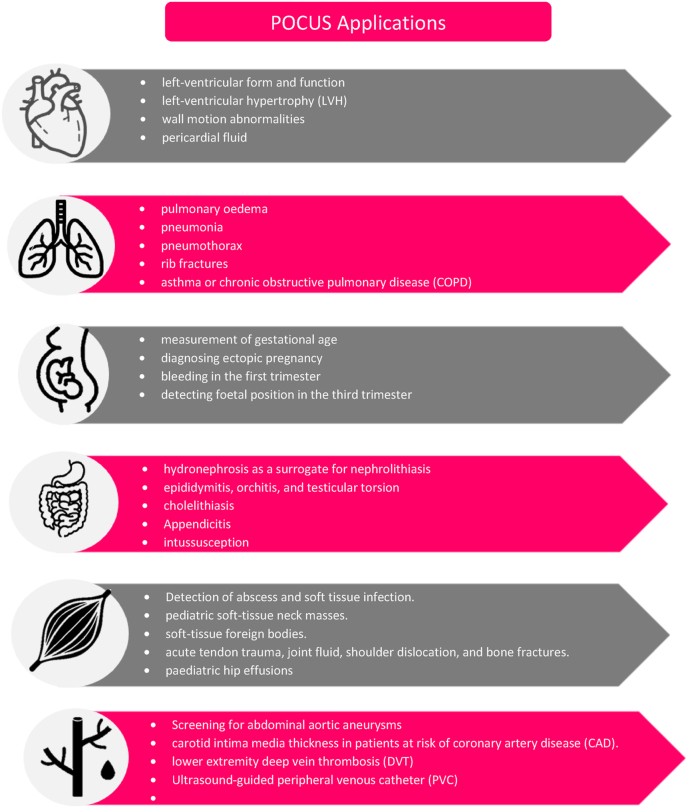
Applications of point of care ultrasound (POCUS) in the cardiovascular, respiratory, obstetrical, gastrointestinal, musculoskeletal, and vascular system.
